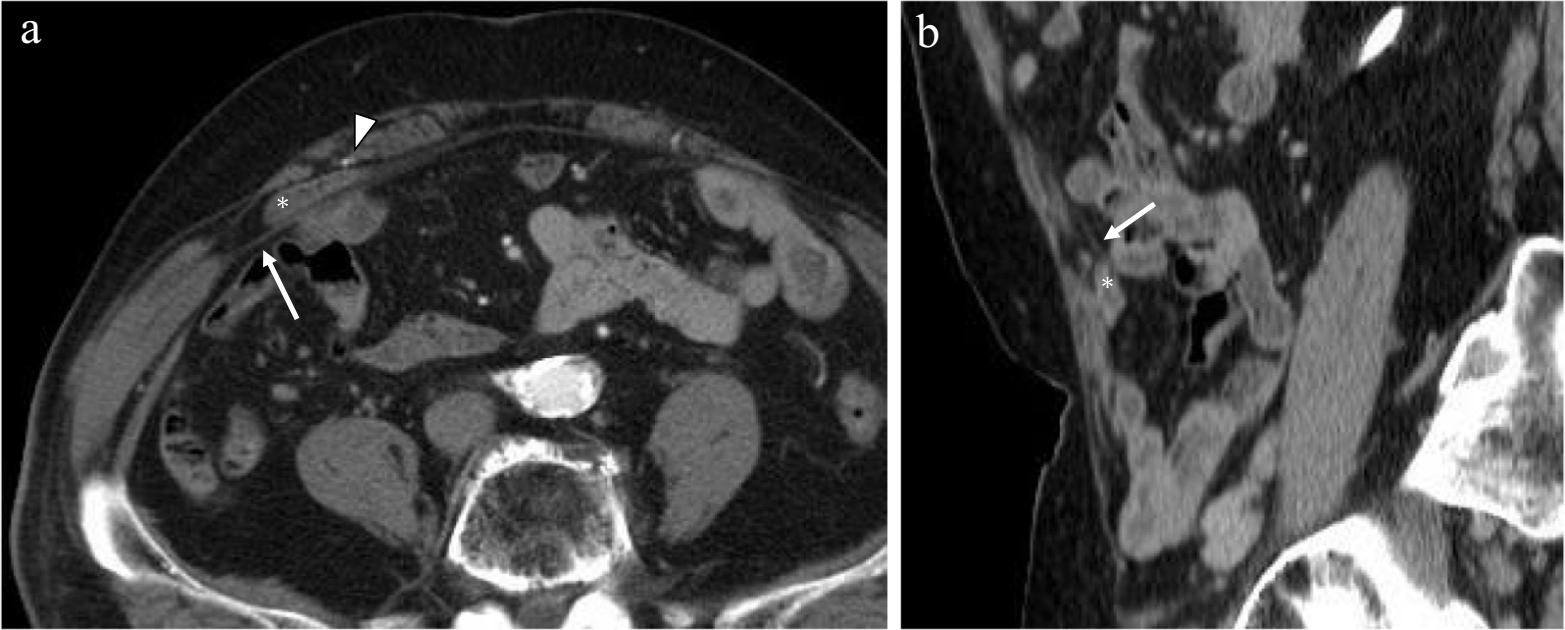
Abdominal contrast-enhanced computed tomography, axial plane (a) and sagittal plane (b). Protrusion of the small bowel (asterisk) is evident between the rectus muscle and the posterior rectus sheath (arrow). The arrowhead indicates the right inferior epigastric artery
